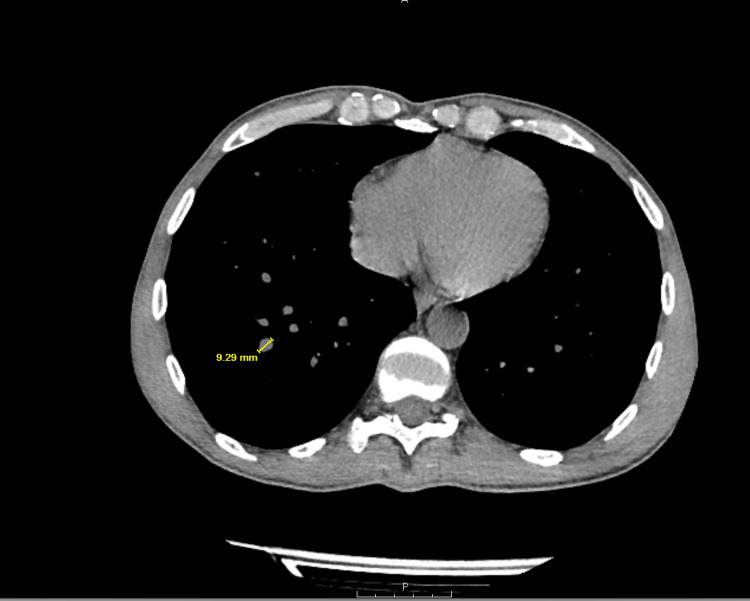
CT chest showing multiple bilateral pulmonary nodules CT of the chest demonstrates multiple bilateral pulmonary nodules concerning for metastatic disease. CT, computed tomography.
